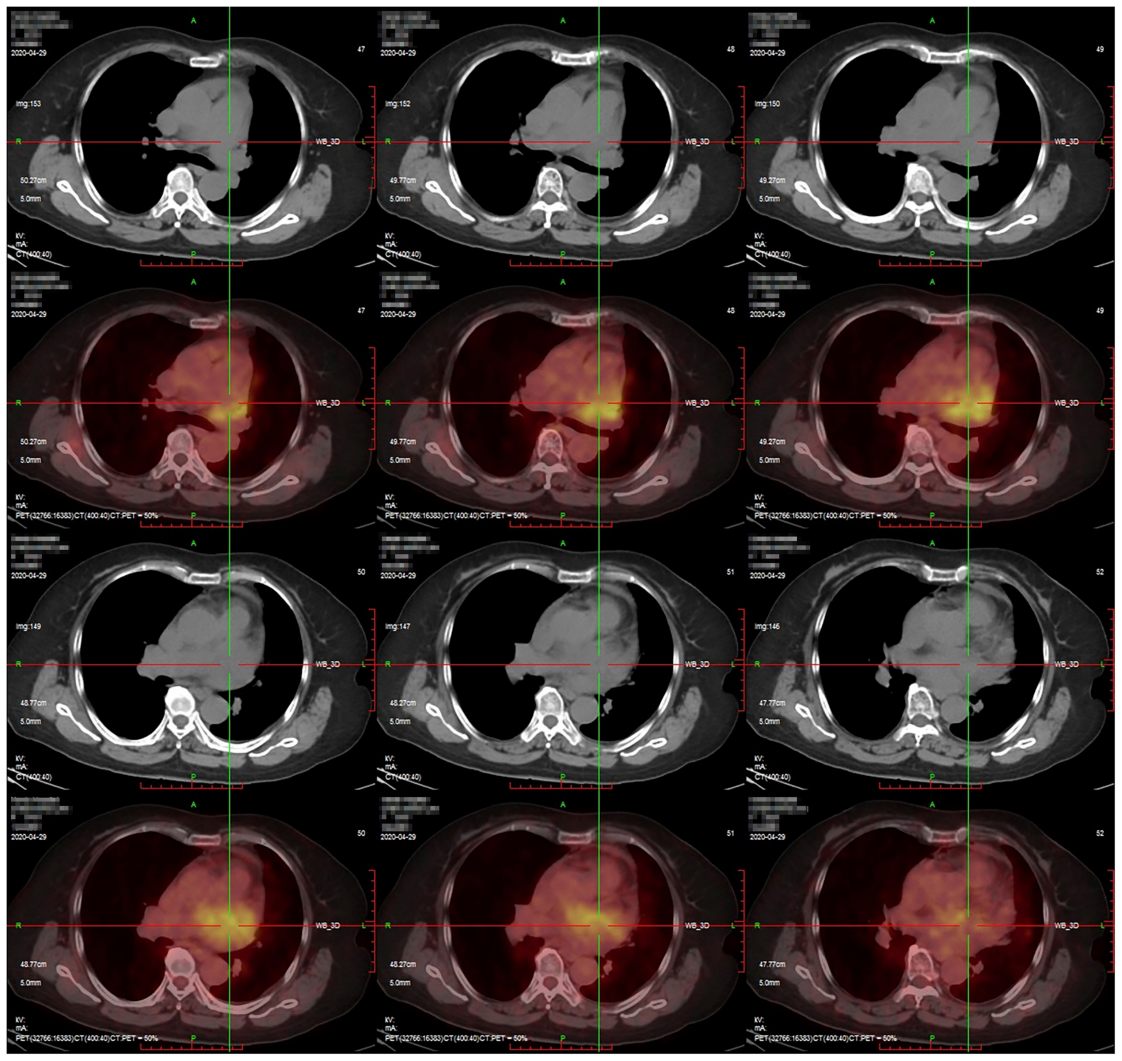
Positron emission tomography/computed tomography findings. Intense fluorodeoxyglucose uptake was observed in the pericardium with a maximum standardized uptake value of 9.6.
